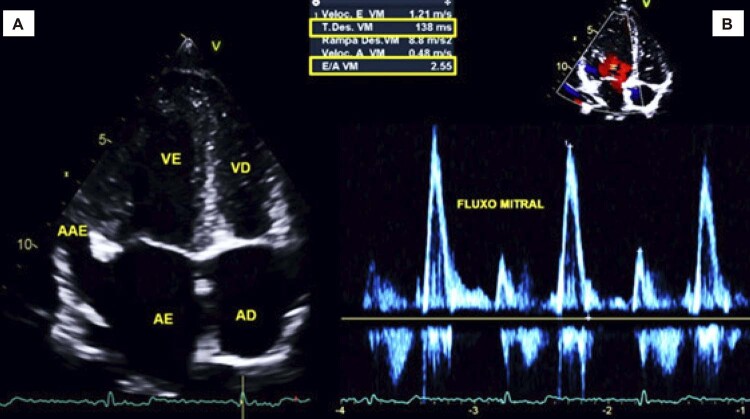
À esquerda corte apical de 4 câmaras ao eco bidimensional. À direta padrão do fluxo mitral ao Doppler em atleta jovem. AAE = apêndice atrial esquerdo; AD: átrio direito; AE: átrio esquerdo; E/A VM: relação E/A da valva mitral; T.Des. VM: tempo de desaceleração da onda E do fluxo mitral; VD: ventrículo direito; VE: ventrículo esquerdo.*
